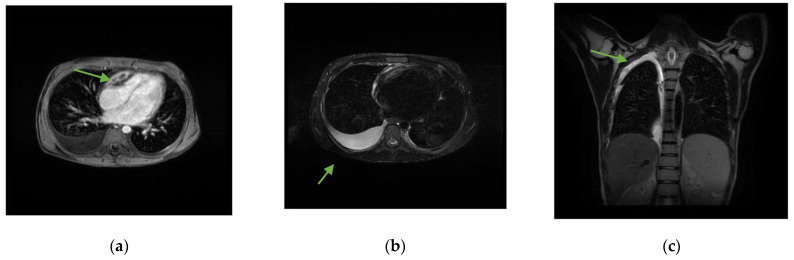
Chest MRI with and without contrast medium. (a) Solid tissue, with some central hypointense areas on T1 and T2 sequences without contrast enhancement, as from fibrosis, located in the upper anterior mediastinal area (arrow) of approximately 6.5 × 2 cm, adherent to the pericardium, which appears thickened in correspondence with the right heart sections, with a consequent compressive effect on the ventricle. (b) Right pleural effusion (arrow) extended to the apex in the supine position (c) with a maximum thickness of approximately 3 cm.
